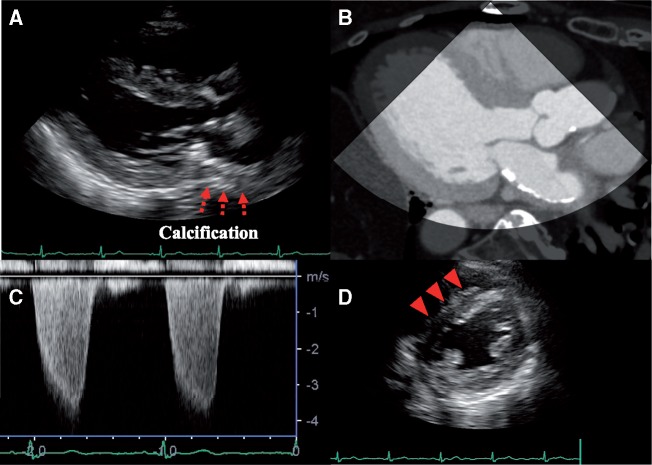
(*A*) Parasternal long-axis view showing a linear high-echoic lesion along the posterior left atrial wall (red arrows), which was demonstrated to be left atrium calcification by multidetector row computed tomography (*B*). A continuous-wave Doppler signal of tricuspid regurgitation is shown (*C*). Peak pressure gradient across the tricuspid valve was measured as 54 mmHg, and configuration of the ventricular septum (red triangle) was distorted to a D-shape in diastole (*D*), suggesting significant pulmonary hypertension.
